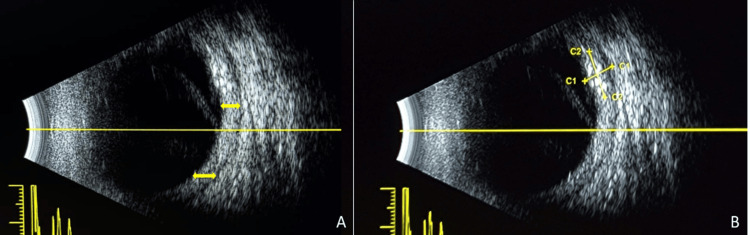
Left eye ultrasound. A. Yellow arrows indicate choroidal thickening. B. Yellow arrows show the dimensions of the mass: 3.27 mm × 4.95 mm.
